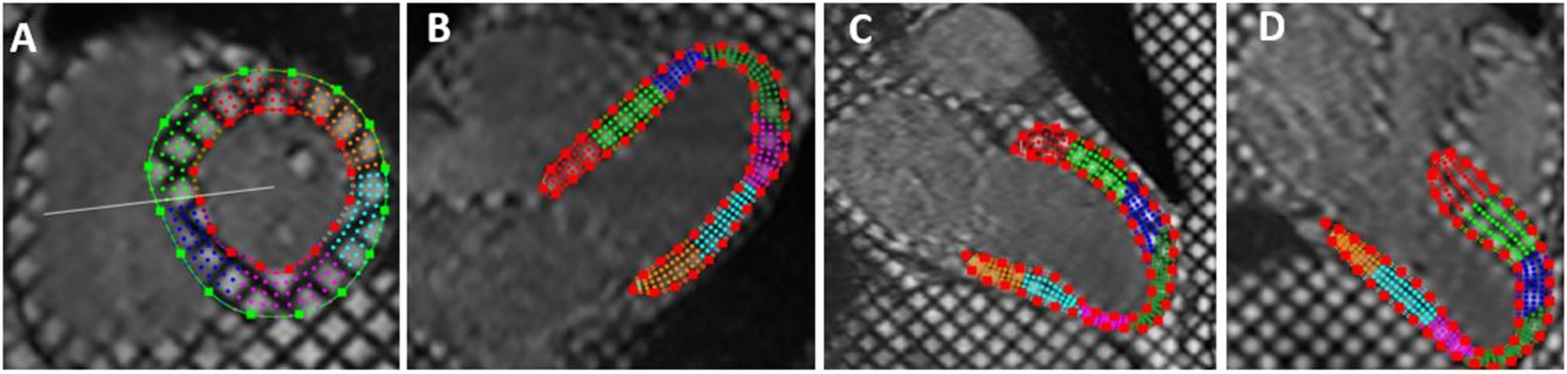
Tagged cardiac magnetic resonance imaging with epicardial and endocardial border tracing in: short axis (A), horizontal long axis (apical four chambers) (B), vertical long axis (apical two chambers) (C), and left ventricular outflow view (three chambers views) (D). The short axis images were used for estimation of circumferential strain while long axis views were used for estimation of longitudinal strain.
